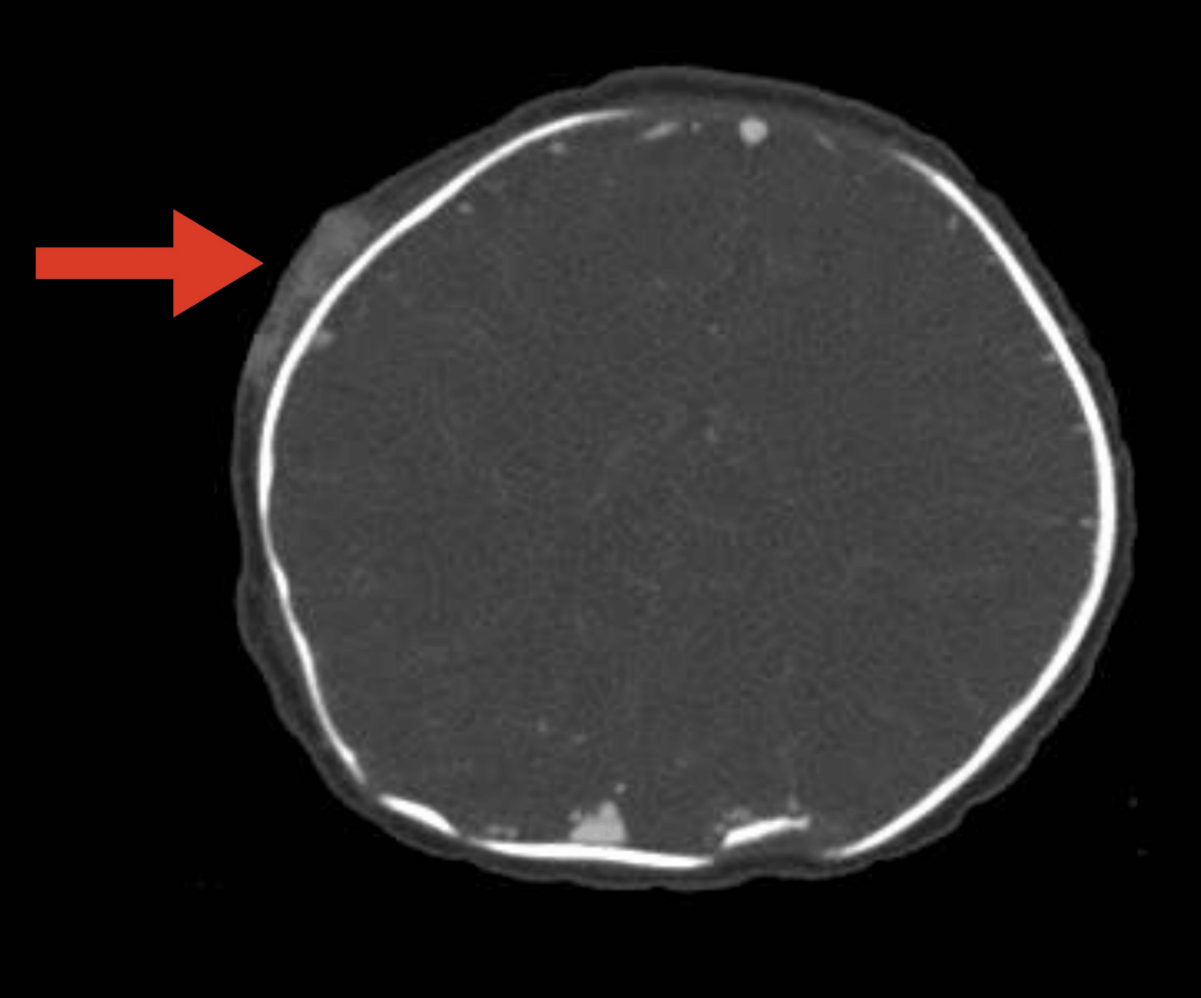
Contrast-enhanced computed tomography showed the mass without bone destruction.
